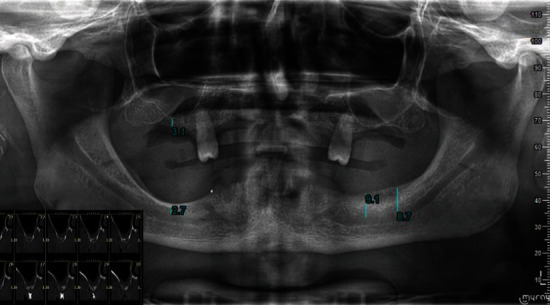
Preoperative orthopantomography and CBCT.
